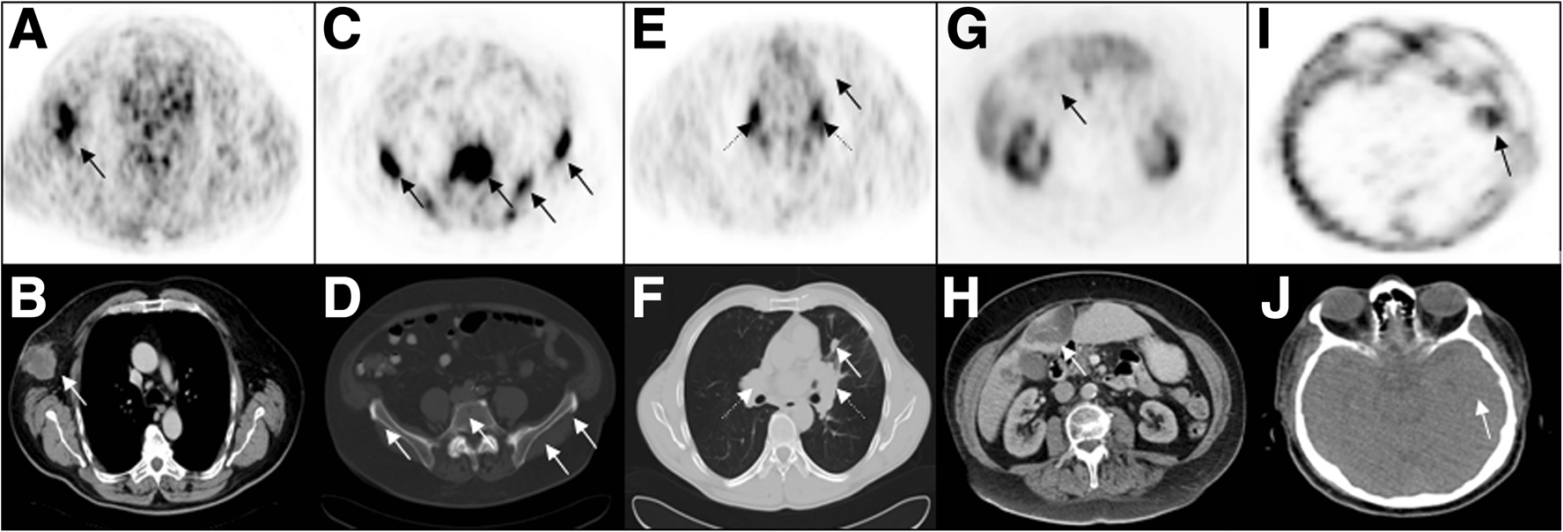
Typical example of SSTR-positive metastases visualized by SSTR-PET. Example of SSTR-positive MCC metastases which were displayed by SSTR-PET imaging. Corresponding CT sections are shown below the transaxial PET views. Whereas lymph node metastases can be visualized with comparable sensitivity (arrows, A, B, E, F), SSTR-PET is more sensitive for detecting bone and brain metastases as compared to CT (C, D, I, J). On the other hand, lung and liver metastases (arrows, E, F) are detected with lower sensitivity by SSTR-PET.
